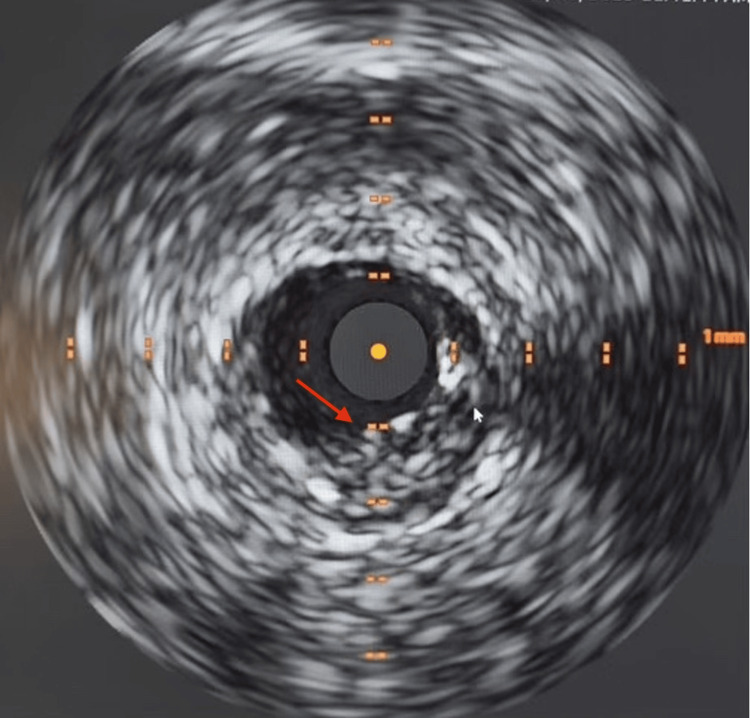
Intravascular ultrasound revealing severe mid-left anterior descending artery stenosis with bulky eccentric plaque burden
